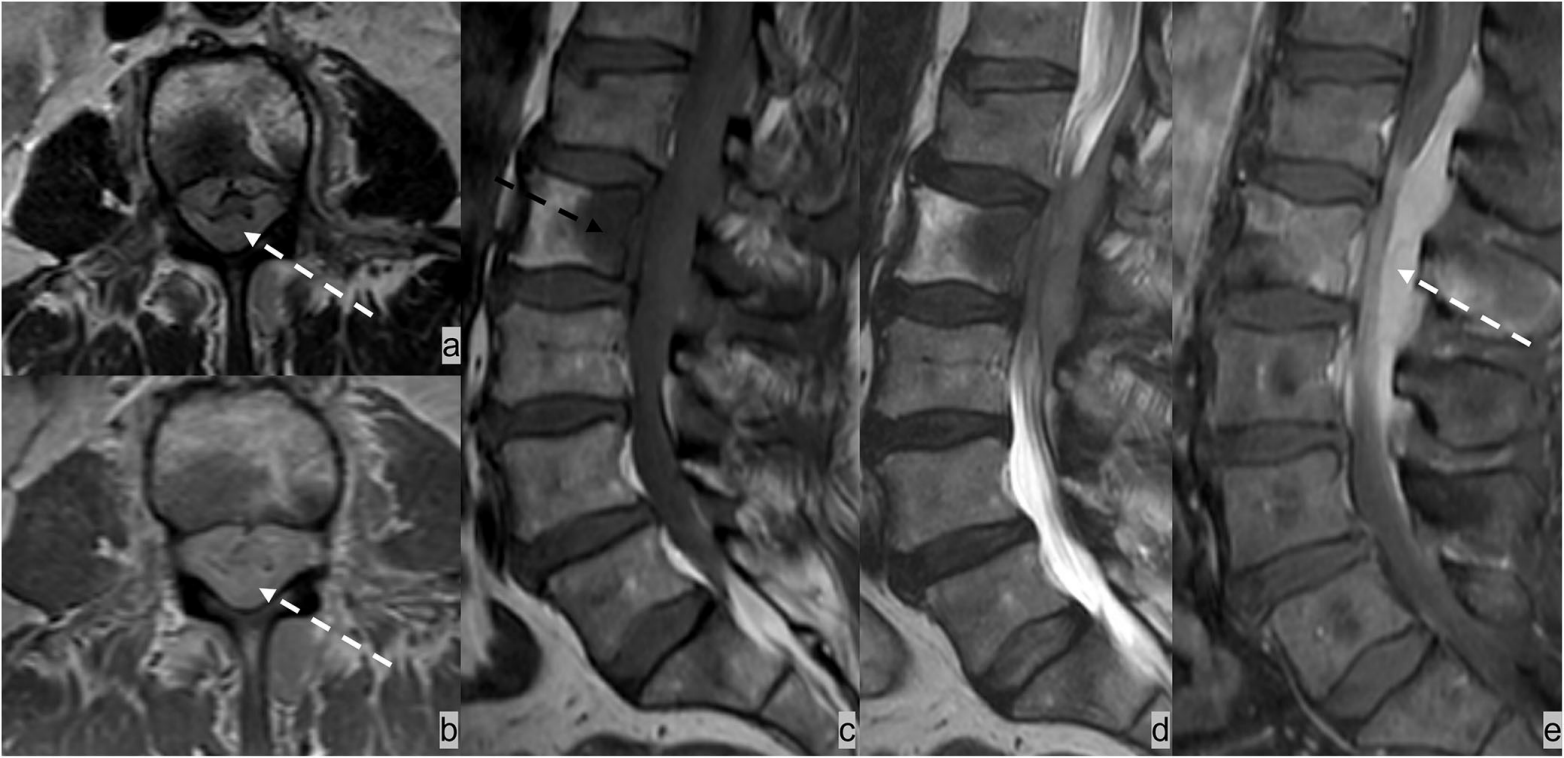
Forty-five-year-old patient presented with cauda equina syndrome including burning pain in the bilateral lower extremities, weakness, and urinary retention. (a) AxialT2w, (b) axial post-contrast T1w, (c) sagittal T1w and (d) T2w, and (e) sagittal post-contrast T1w MRI sequences demonstrated an enhancing T1 hypointense and T2 iso-hypointense osseous lesion involving L2 vertebrae (dotted black arrow) and an enhancing epidural soft tissue completely encasing the thecal sac and compressing cauda equina nerve bundles (dotted white arrow). Biopsy of the L2 lesion showed germinal center-type diffuse large B cell lymphoma.
